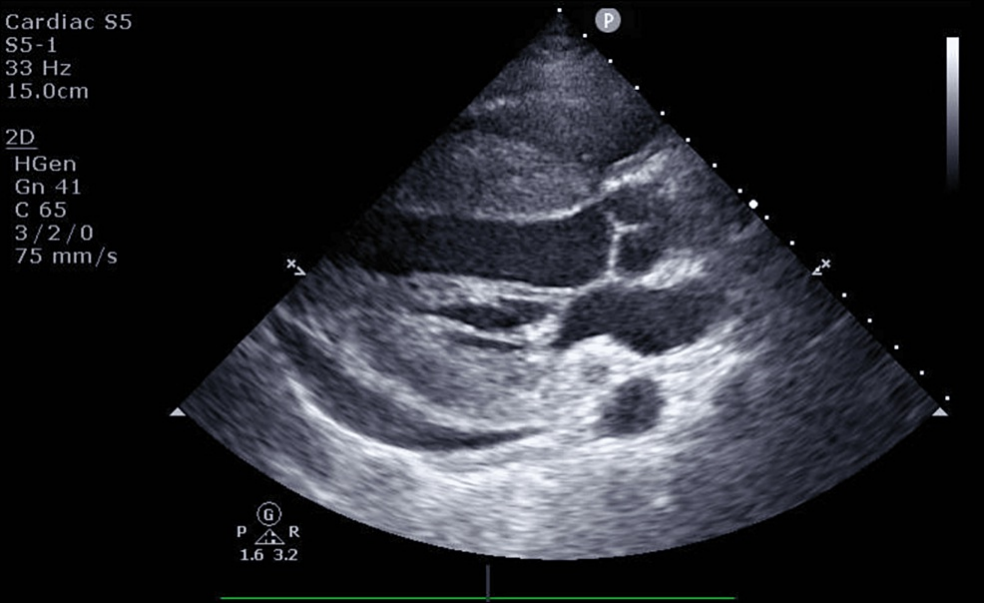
Transthoracic echocardiography Echocardiography at the time of exacerbation of myocarditis showed left ventricular hypertrophy and pericardial effusion.
